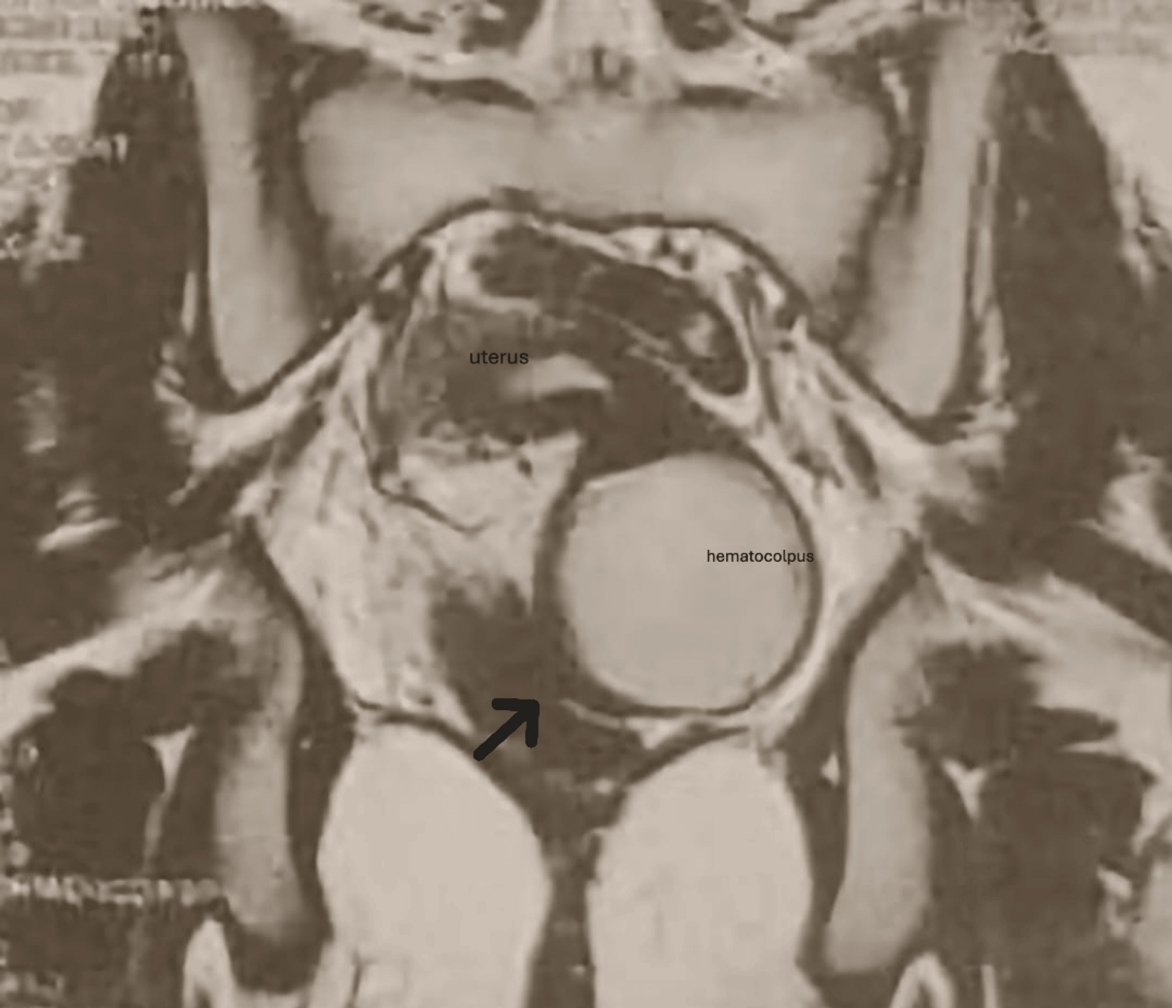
Pelvic MRI, T2-weighted coronal sequence, demonstrating hematometrocolpos with proximal vaginal distension The arrow indicates distal vaginal stenosis located downstream from the hematocolpos, consistent with distal vaginal atresia.
